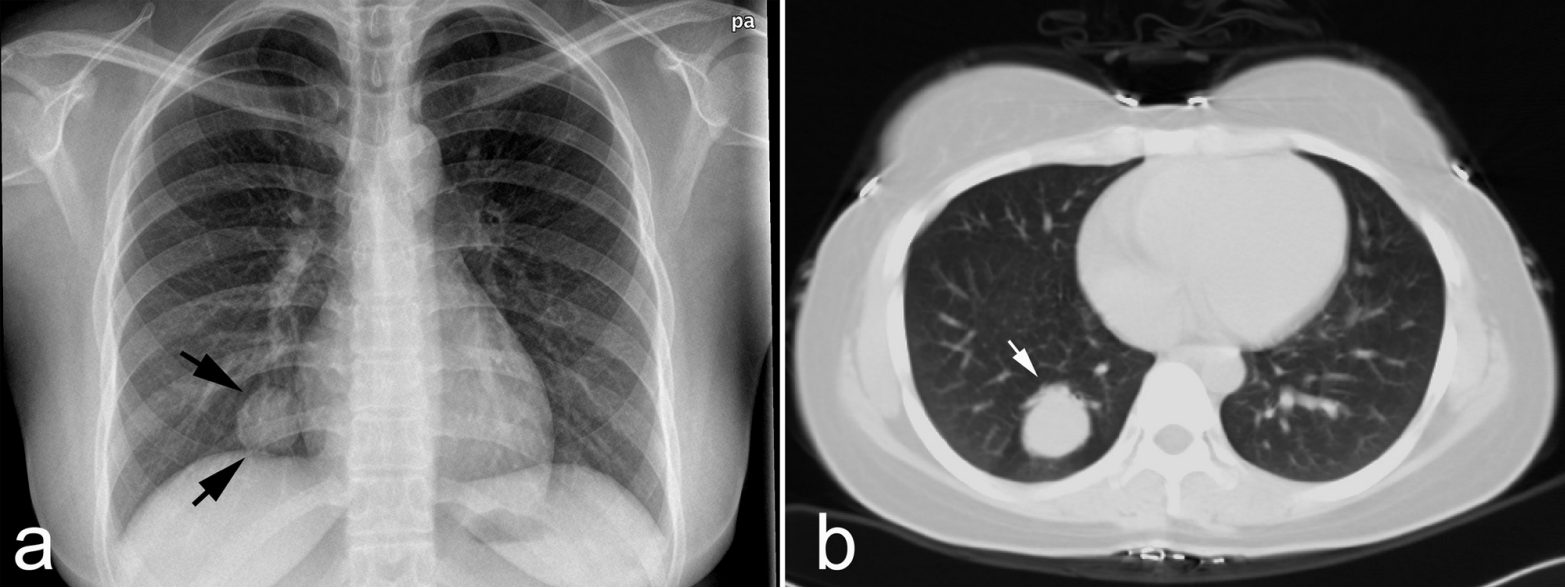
Preoperative chest radiology of lesion. (a) Chest X-ray revealed solitary pulmonary mass in right lower lung field (black arrow). (b) Chest CT image showed well-defined mass in right lower lobe (white arrow). There was no remarkable image finding was noted in the surrounding lung parenchyma.
